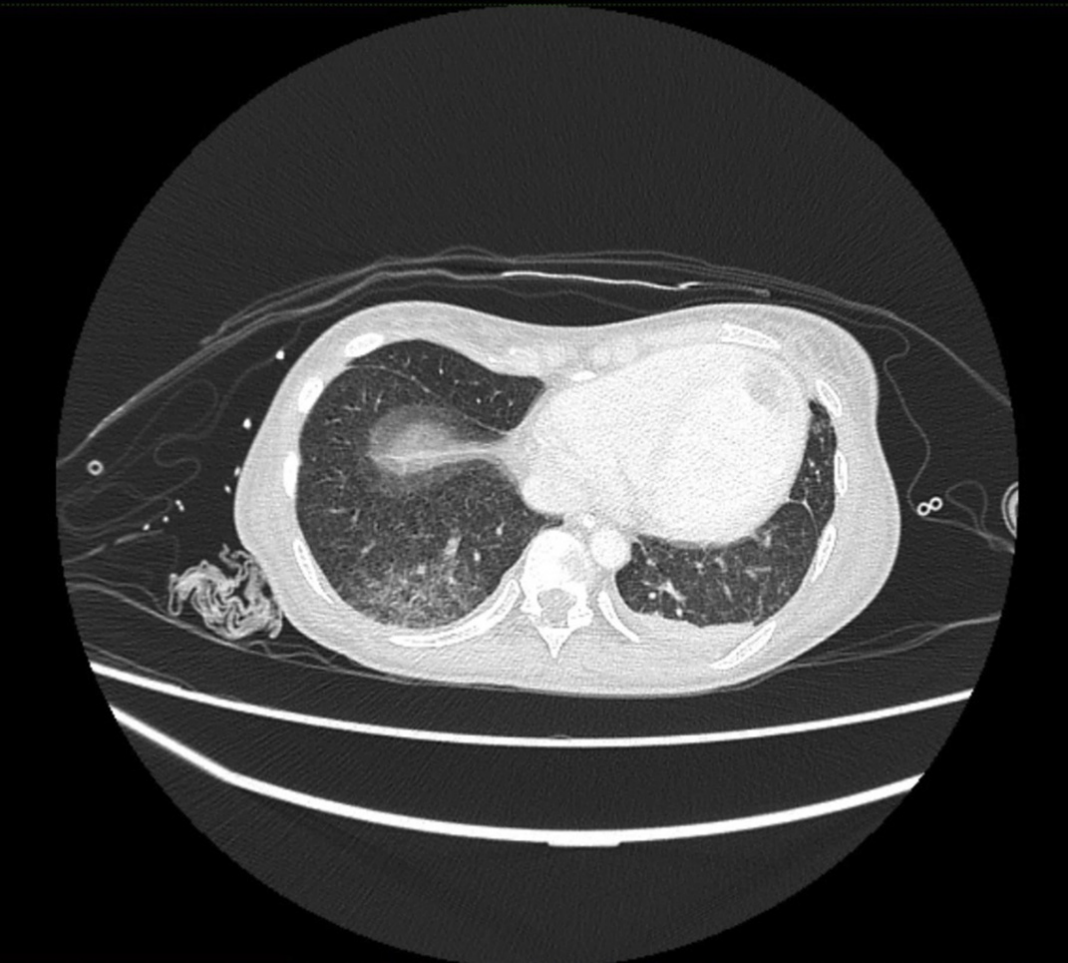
Chest Computed Tomography Showing Axial View of the Left Ventricular Apical Thrombus
